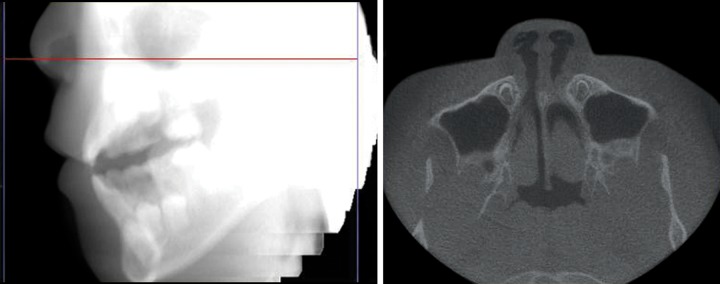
CBCT scan image revealed normal mucosal thickness and no opacification of the right maxillary sinus 2 months after the removal of needle
